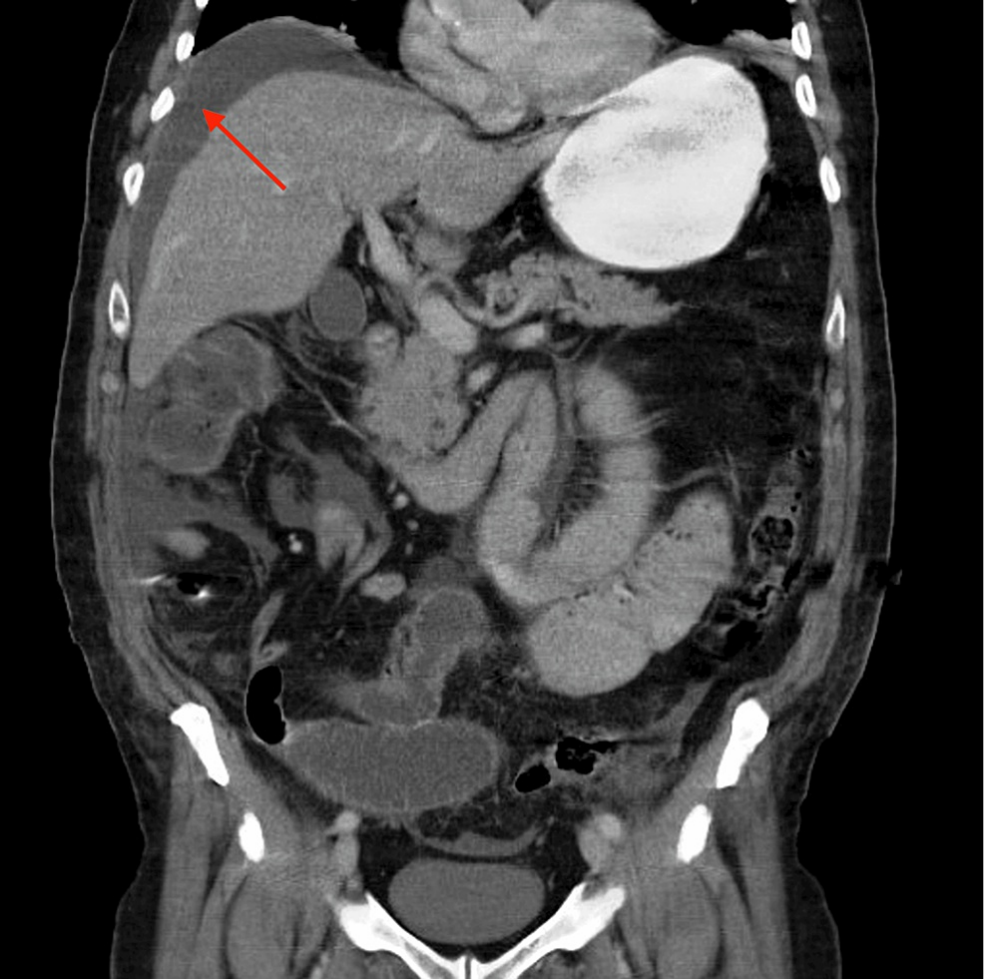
CT Abdomen and Pelvis Free intraperitoneal collection located between the liver and the diaphragm.
